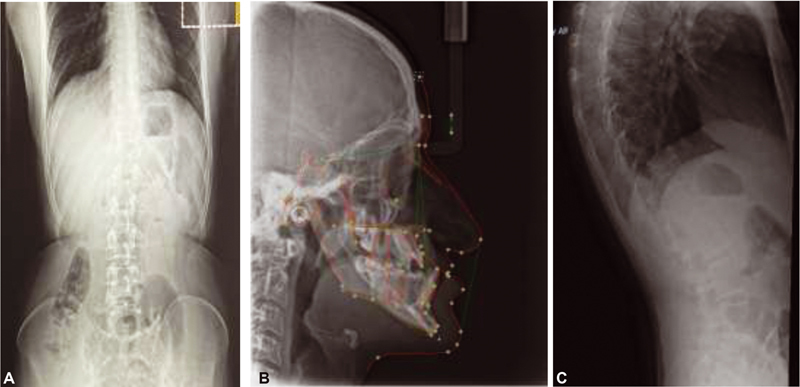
Radiological characteristics (A–C) radiographs of patient maintained thoracic kyphosis and lumbar lordosis. Diffuse decreased bone density; osteoporosis. Decreased height of T-7, 8, 9, and T-12 vertebral bodies. Normal height and alignment of the other vertebral bodies. C lateral cephalometric showed severe Cl III malocclusion due to combination between retrognathic maxilla and prognathic mandible.
